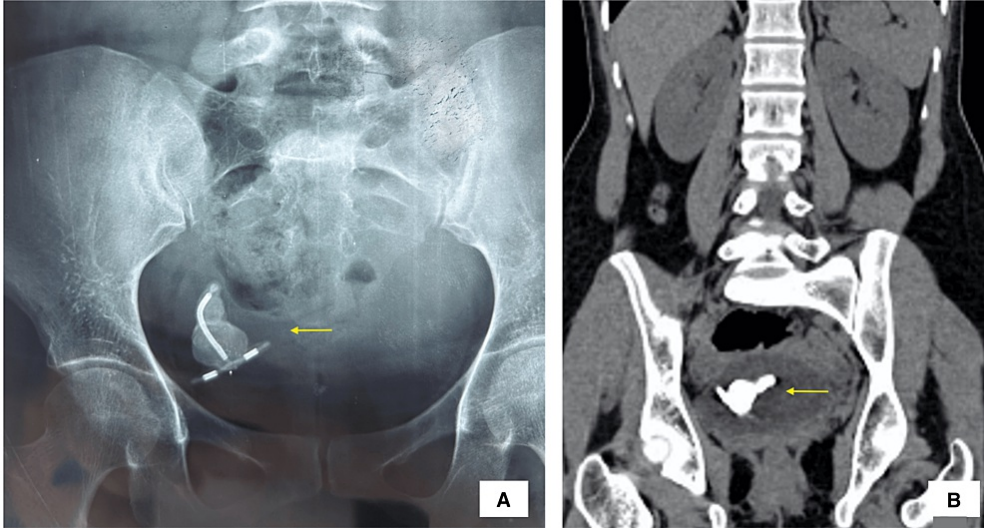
Radiological images (X-ray (A) and computed tomography scan (B)) showing the migrated IUD Arrow: Encrusted intravesical IUD IUD: intrauterine device
